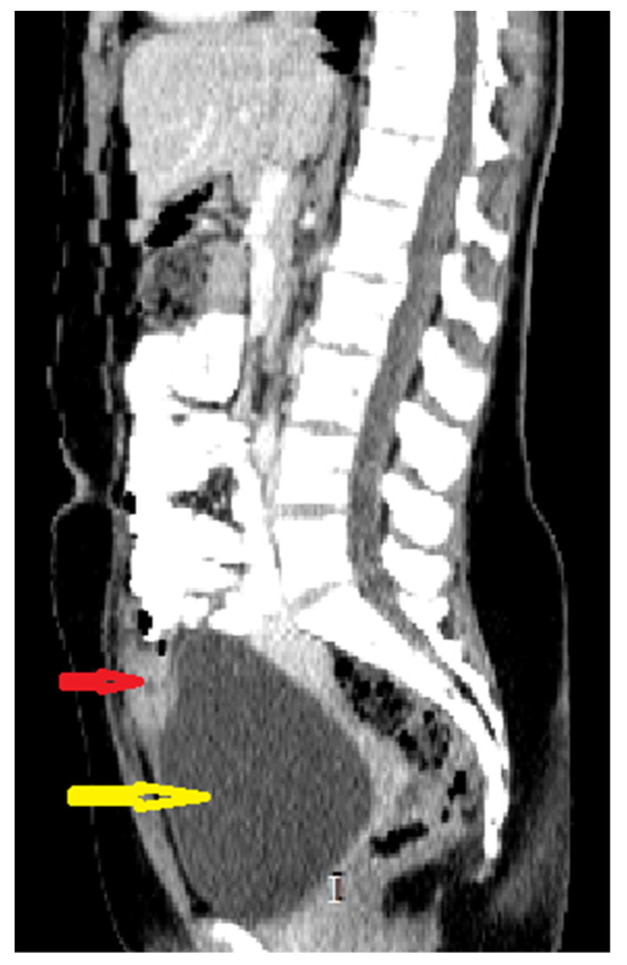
Sagittal CT scan reconstruction of abdomen and pelvis with intravenous and oral contrast: red arrow: supposed urachal cyst; yellow arrow: urinary bladder.
